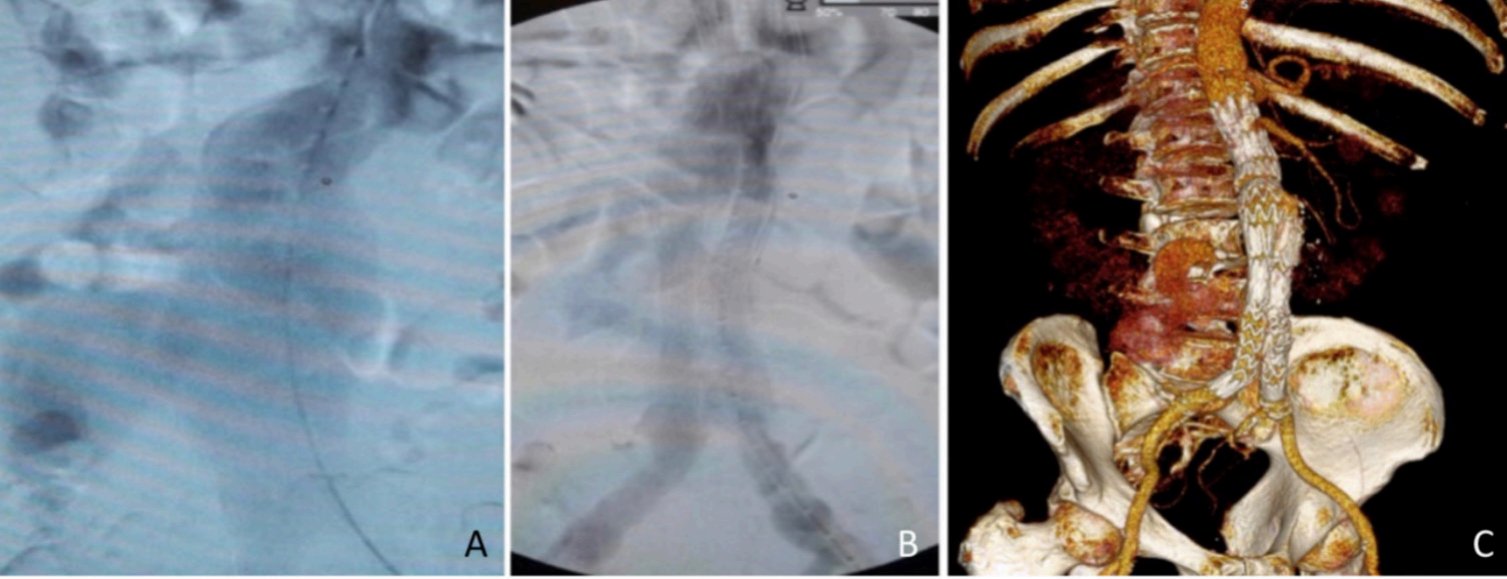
Case#2 (A) Intraoperative DSA showing the RAAA involving an AF, demonstrated by early filling of the inferior vena cava; (B) Intraoperative DSA after endograft deployment; (C) Postoperative 3D-CTA showing complete AF resolution and adequate sealing. AF = Aortocaval fistula; DSA = digital subtraction angiography; RAAA = ruptured abdominal aortic aneurysms; 3D-CTA = three-dimensional-by-volume CT angiography.
